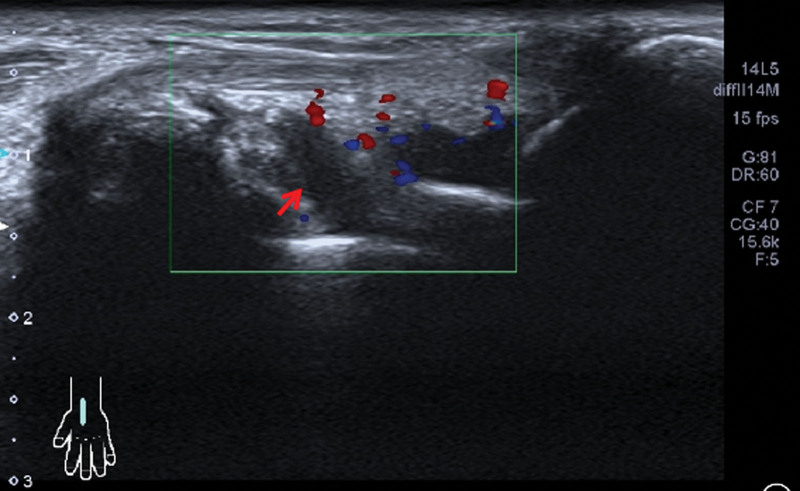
Ultrasound images of the patient wrist. Arrows showed synovial thickening of the synovium of both wrists and a small amount of blood flow signal.
